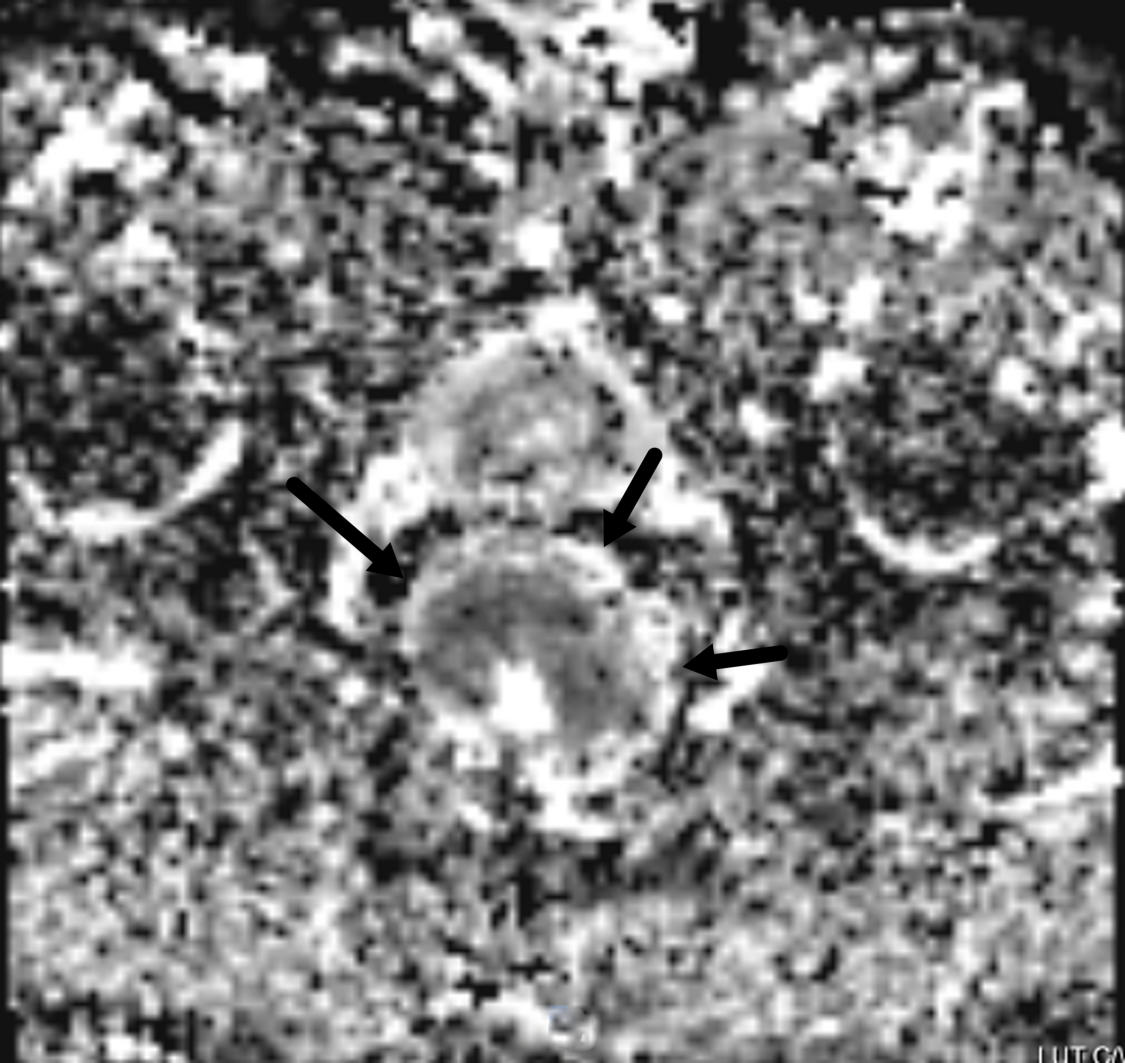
An MR image of a rectal carcinoma. On the MD map, the lesion is the low signal.
